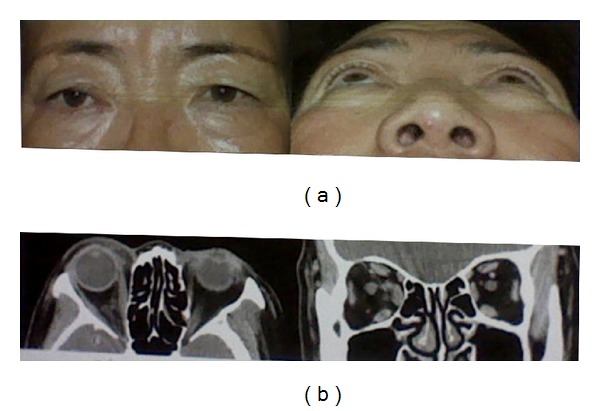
(a) Postoperatively, photographs showing that proptosis is improved with good cosmesis. (b) Postoperative CT scan demonstrating the complete removal of the tumors.
